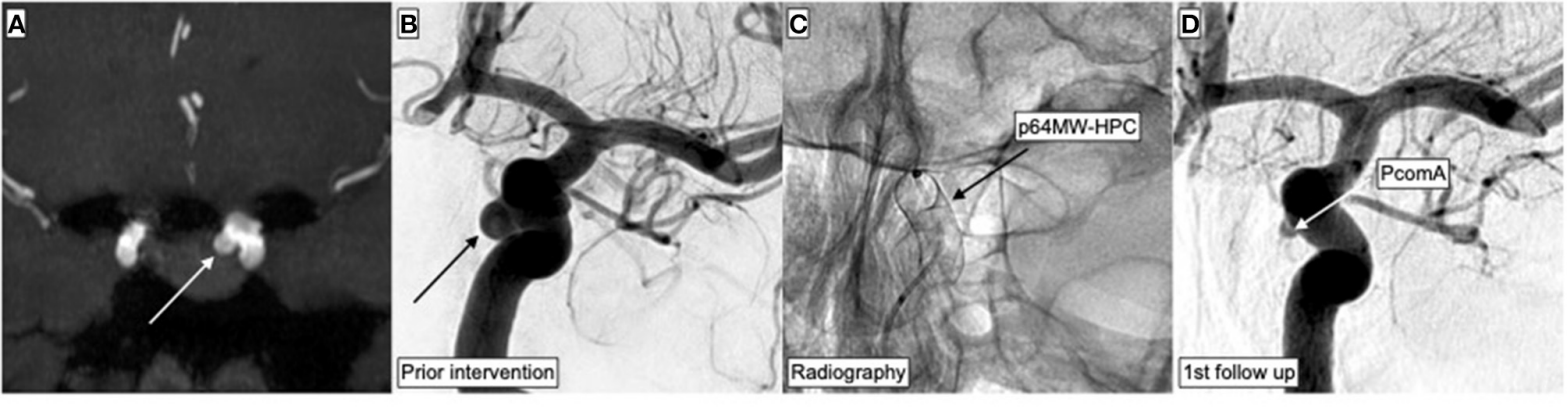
Endovascular treatment of an incidental aneurysm arising from the left-handed side ICA. MRI was performed as preoperative imaging for planning of pituitary adenoma surgery. The growth-hormone-secreting adenoma had caused acromegaly. After detection of the aneurysm, decision was made for preceding flow diversion in order to facilitate future transsphenoidal resection without increased risk for arterial bleeding. (A) TOF-angiography maximum intensity projection (MIP) demonstrates the 4.5-mm measuring ICA aneurysm and its anatomical relationships. (B) Corresponding DSA in posterior–anterior projection demonstrates the aneurysm (black arrow) prior to treatment. (C) Radiography immediately after implantation of the p64MW-HPC in a posterior–anterior projection, matching (B,D). (D) First follow-up DSA after 3.5 months in posterior–anterior projection; the aneurysm is completely occluded.
